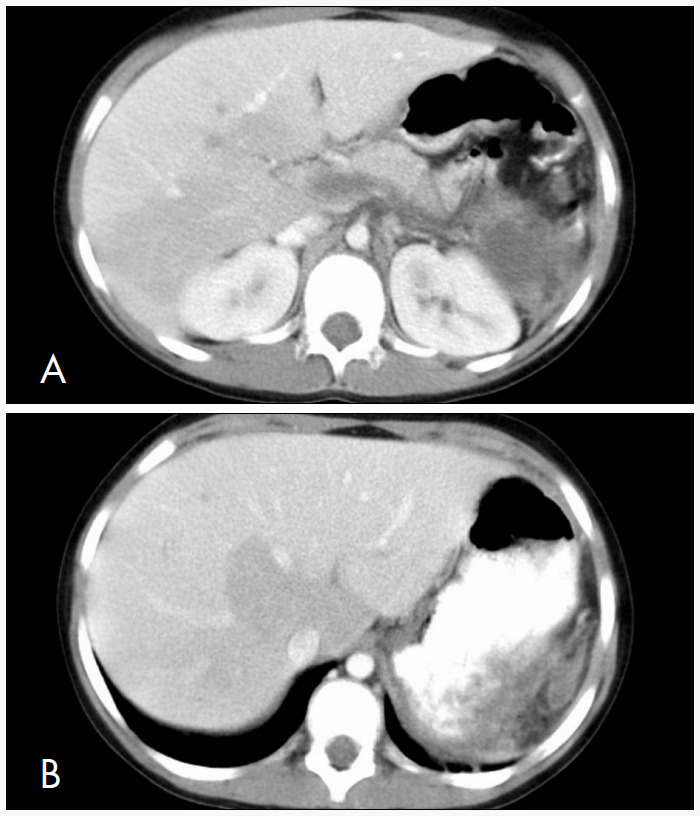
Abdominal computed tomography scan on the eighth postoperative day following laparoscopic splenectomy, showing thrombosis of the splenic vein, main portal vein, right and left portal veins, and portions of the smaller intrahepatic portal vein branches.
